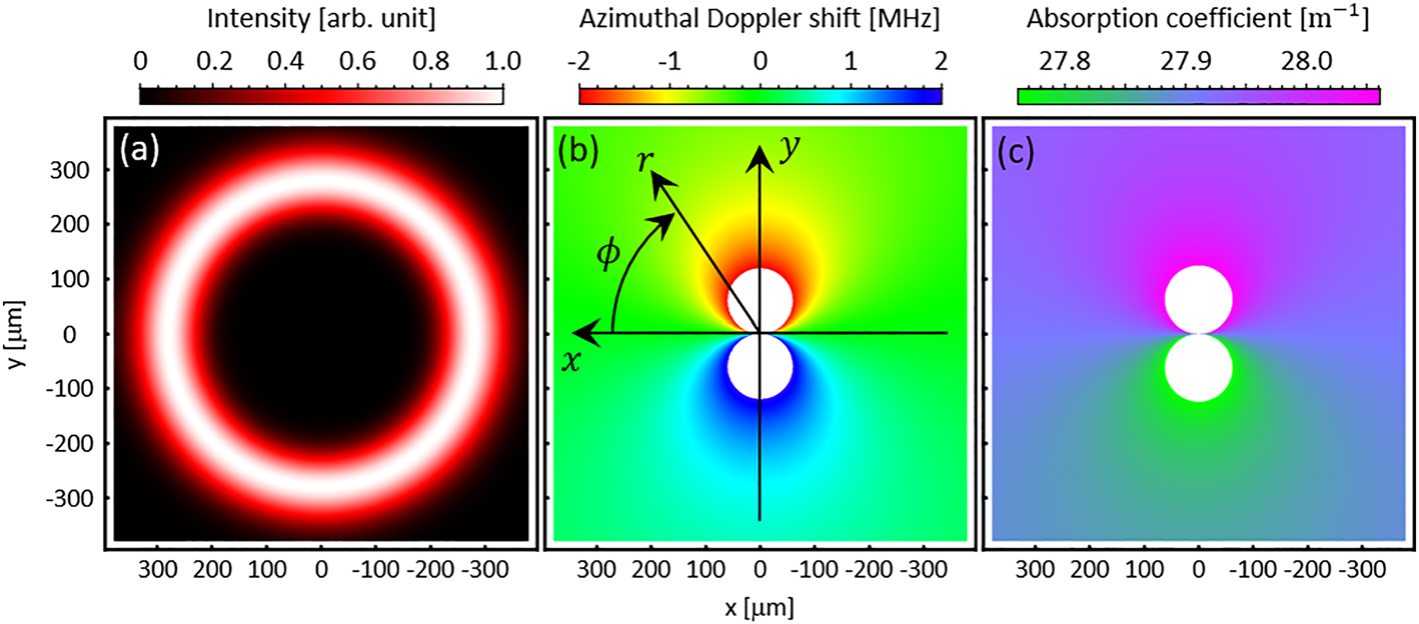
Numerical calculation results for (a) normalized intensity distribution for optical vortex beam, (b) azimuthal Doppler shift distribution, and (c) absorption coefficient distribution. The calculations are performed with parameters $$\ell =+10$$, $${U}_{x}=150$$ m/s, and detuning = 360 MHz.
